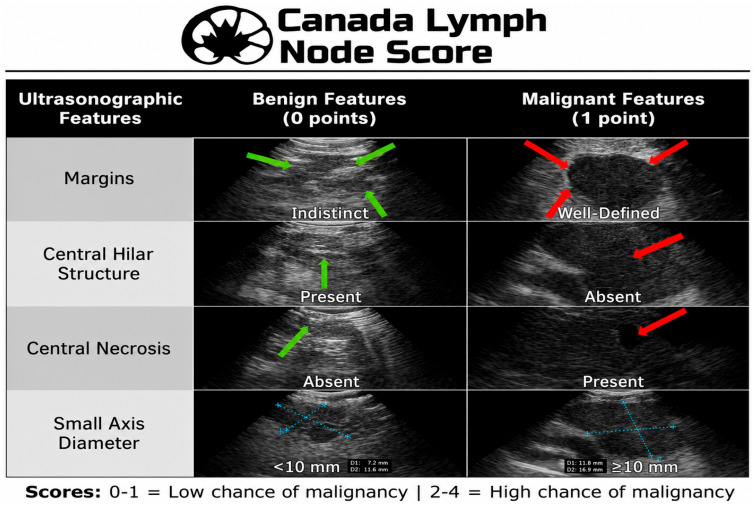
Canada Lymph Node Score for ultrasonographic assessment of mediastinal lymph nodes. The scoring system is based on four sonographic features: nodal margins (indistinct vs. well-defined), central hilar structure (present vs. absent), central necrosis (absent vs. present), and small axis diameter (<10 mm vs. ≥10 mm). Each malignant feature scores 1 point, with total scores ranging from 0 to 4. A score of 0–1 indicates a low probability of malignancy, whereas a score of 2–4 suggests a high probability of malignancy. (copyrights license 6266321270801).
